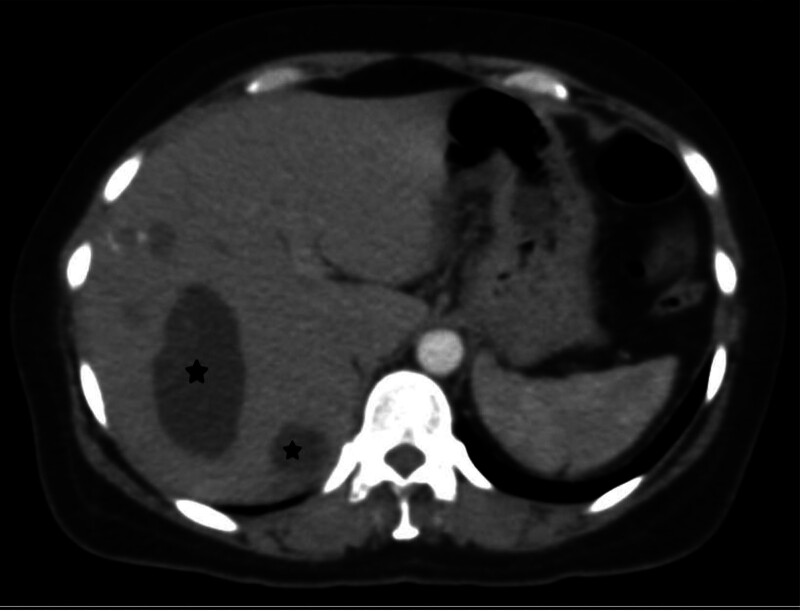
An axial contrast-enhanced computed tomography (CT) scan demonstrates the presence of hepatic hydatid cysts located in the right lobe of the liver (black stars). CT = computed tomography.
